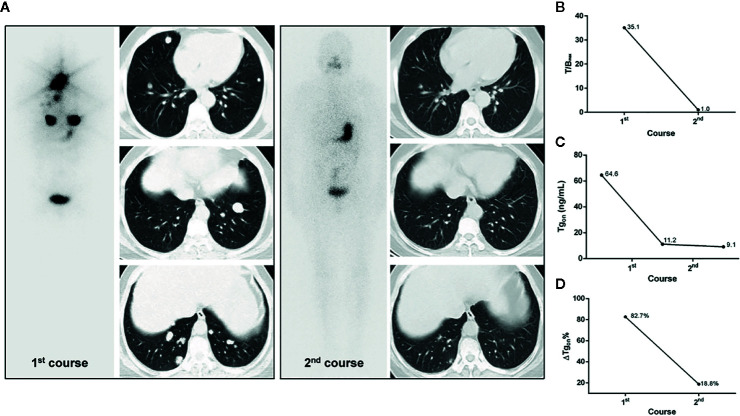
A 53-year-old female differentiated thyroid cancer patient with robust ^131^I-avid pulmonary metastases achieving excellent benefit from the initial RT. The T/B_max_ and ΔTg_on_% resulting from the initial course of RT were 35.1 and 82.7%, respectively. In the second course of RT, unexpectedly, post therapeutic whole body scans were negative and the size of lesions were dramatically decreased on chest computed tomography, along with a Tg_on_ of 9.1 ng/ml, indicating an excellent benefit from the initial course of RT, which was demonstrated by a continuous decrease in Tg_on_ to 0.9 ng/ml during the 3-year follow-up. (A) Whole body scans (anterior) at 3 days after the oral administration of 7.4 GBq of ^131^I; (B) T/B_max_; (C) Tg_on_; (D) ΔTg_on_%. RT, radioiodine treatment; T/B_max_, maximum target-to-background ratio; Tg_on_, thyroid stimulating hormone-suppressed thyroglobulin; ΔTg_on_%, change rate of Tg_on_ level.
